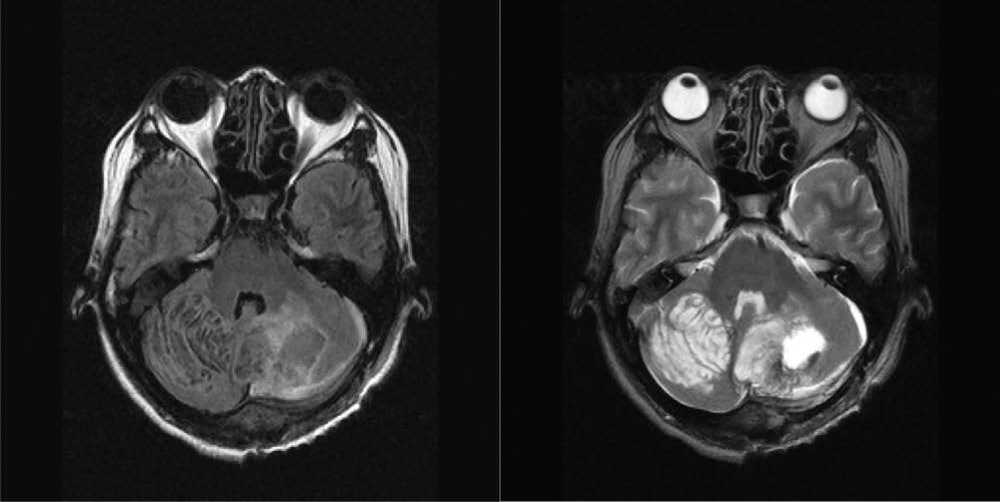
Magnetic resonance images of bilateral cerebellar hamartomas in the patient aged 40 years, after left tumor resection. Left: T1-weighted image, right: T2-weighted image.
